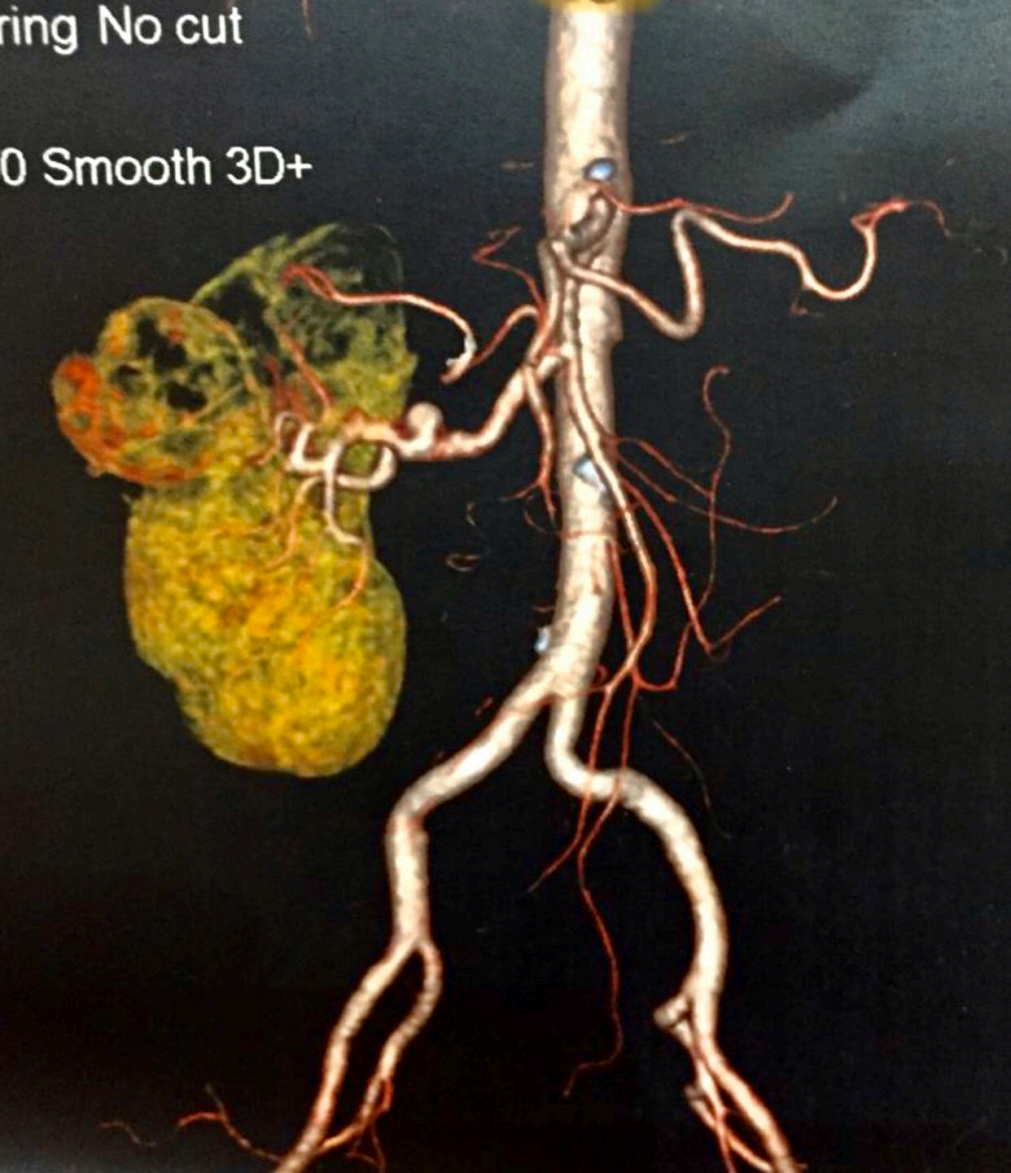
Preoperative computed tomography reconstruction showing tumorous lesion and renal artery aneurysm.
